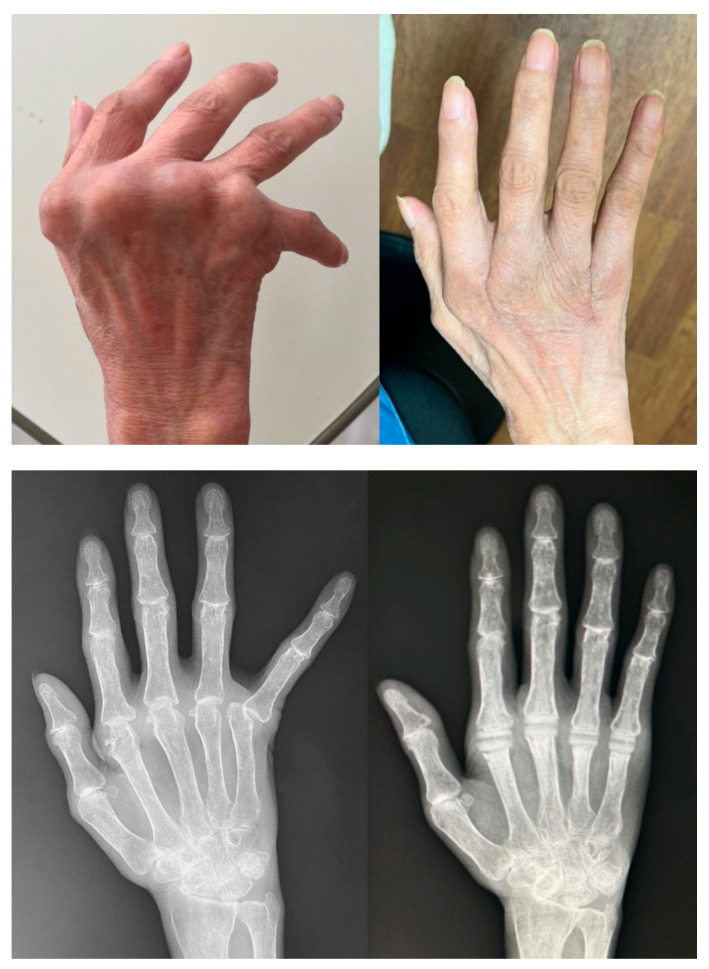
Preoperative and postoperative appearance and radiographs for MCP joint replacement with silicone implants ((left): preoperative; (right): postoperative). Ulnar deviation is improved both in appearance and in the radiographs.
